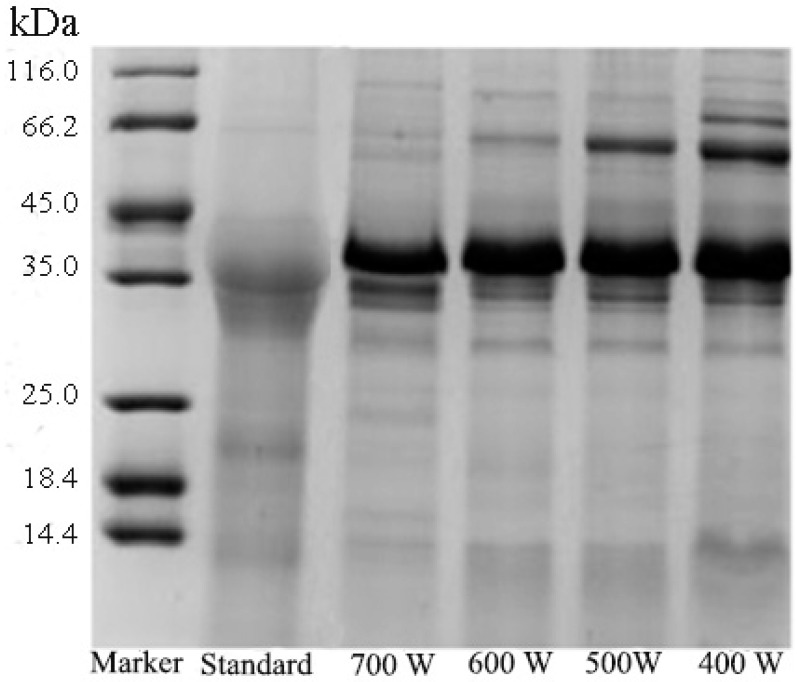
SDS-PAGE of Pv for different ultrasound powers (400, 500, 600, and 700 W). Other operating conditions: Heating temperature, 80 °C; heating time, 15 min; and ultrasound time, 10 min.
